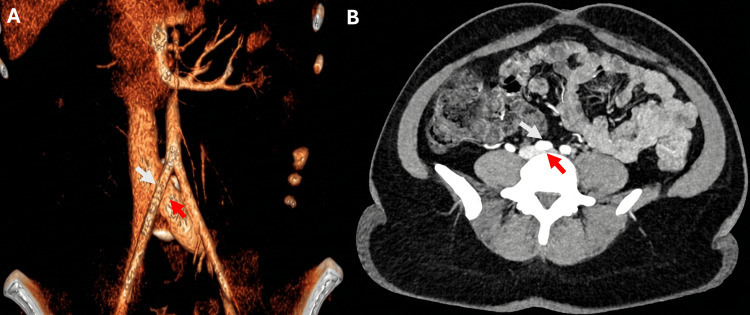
Abdominal computed tomography angiography showing compression of the left common iliac vein (red arrow) by the right common iliac artery (white arrow) on coronal (A) and axial (B) views.
